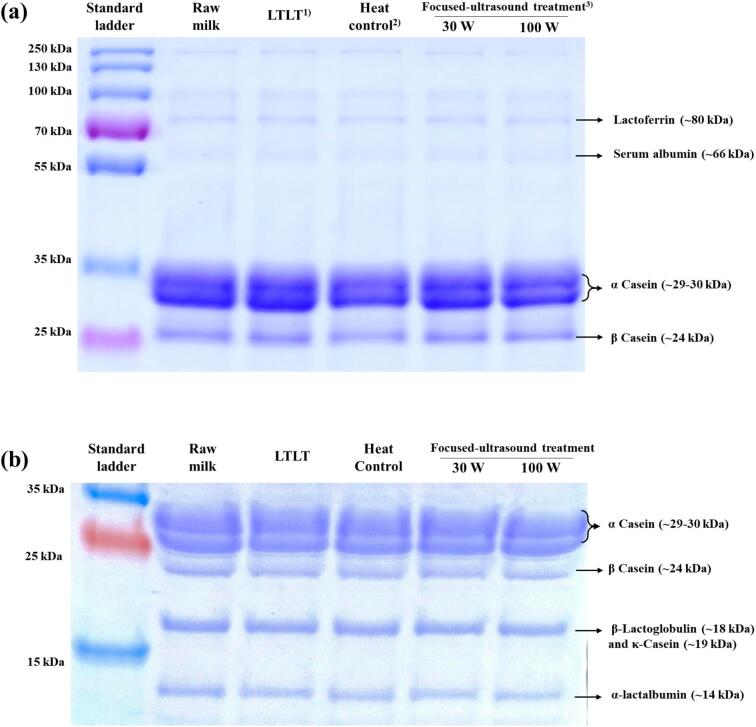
Electrophoretic banding patterns obtained under SDS-PAGE in milk treated with focused ultrasound. (a) 15 % separating gel; (b) 16 % separating gel. ^1)^Raw milk heated at 63 °C for 30 min; ^2)^Raw milk heated at 55 °C for 30 min; ^3)^Raw milk treated with focused-ultrasound at 55 °C for 30 min.
